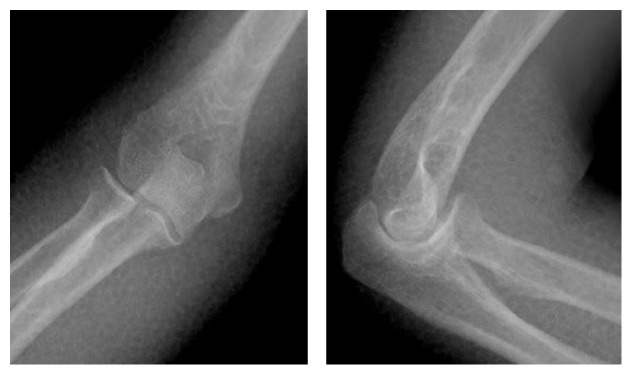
Postreduction radiographs revealed no fractures.
